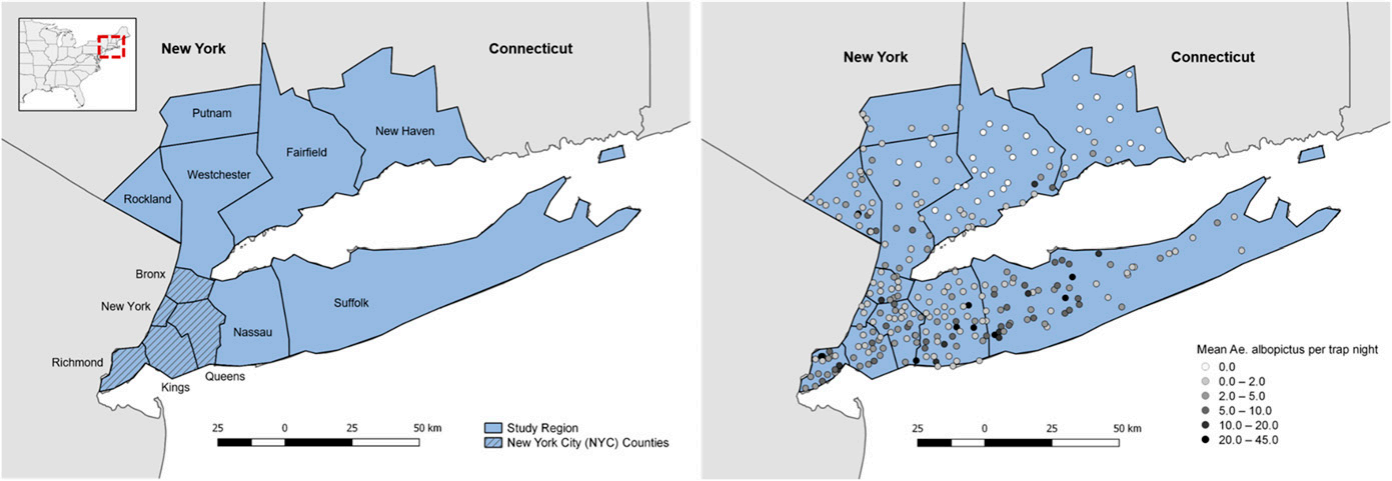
Map of the study region, New York state (NYS) and Connecticut (CT), United States. (A) Counties included in the study region. Ten counties in NYS (Suffolk, Nassau, Queens, Kings, Richmond, New York, Bronx, Westchester, Rockland, and Putnam) and two in Connecticut (Fairfield and New Haven). Five NYS counties make up New York City (NYC) and include Queens, Kings, Richmond, New York, and the Bronx; these correspond to the boroughs of Queens, Brooklyn, Staten Island, Manhattan, and the Bronx, respectively. (B) Distribution of trap sites (*N* = 332) throughout the study region. During May–September 2017, *Aedes albopictus* mosquitoes were present in approximately 89% of sites (*N* = 297). This figure appears in color at www.ajtmh.org.
